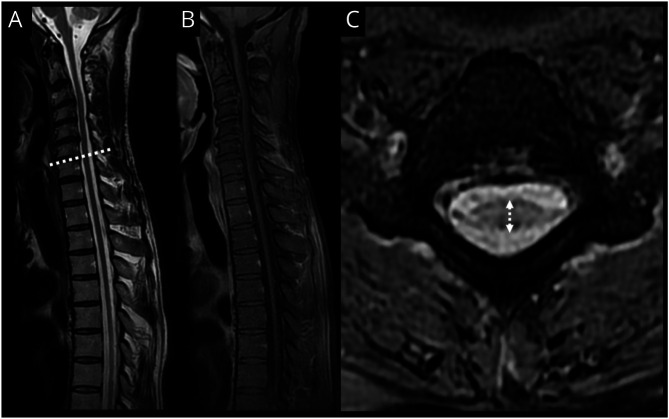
Spinal MRI in Chronic HAM Sagittal T2w and postcontrast, fat-saturated T1w (A and B) and axial T2w (C) MR images at the level of the cervicothoracic junction in a patient with chronic HAM demonstrates long-segment diffuse spinal cord volume loss greatest in the anteroposterior dimension.
